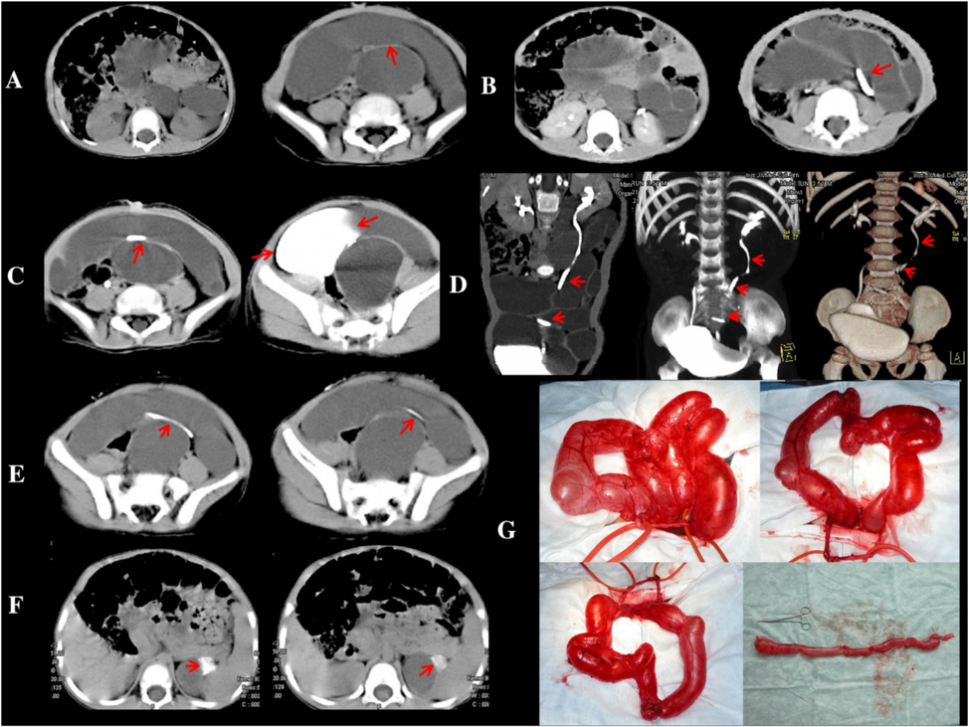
Case 3. CT obtained in a 3.5-year-old boy with left duplex kidney & ureters, and congenital megaflop-ureter. Non- enhanced scan obtained at the abdomen & pelvis shows fluid-filled loops, the normal bowel loops are compressed to the right side of the abdominal wall, and a tendon-like shadow can be seen in the bowel loop-like areas (red arrow) (a). The tendon-like shadow was intensified as the normal ureter (red arrow) (b), and the normal bladder was also intensified in the left side pelvis (*red arrow*) on the 54-min scan (c). Meanwhile, MIP reconstruction shows the position and course of left ureter changed (*red arrow*) (d). The left ureter shows delayed enhancement on the 3-h and 13-min scan (e). The area of the left side kidney shows a little irregular enhancement on the 23 h and 9-min scan (*red arrow*) (f). Operation findings (g)
